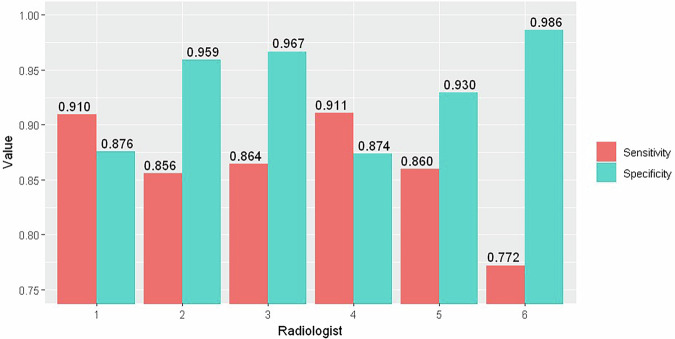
Diagnostic performance of six B readers in detecting TB on CXRs. Sensitivity and specificity are shown for each reader. CXR, chest X-ray
